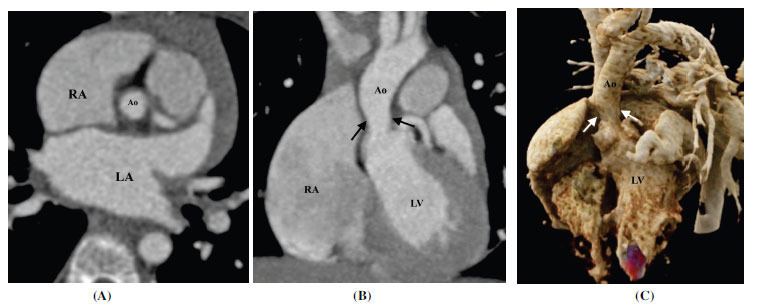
Supravalvular aortic stenosis. (A) Axial contrast-enhanced computed tomographic angiography image showing the narrowed caliber of the supravalvular aorta (B) Coronal contrast-enhanced computed tomographic angiography image and (C) Volume rendered technique image showing supravalvular aortic stenosis (arrow). Abbreviations: RA; right atrium, LA; left atrium, LV; left ventricle, Ao; ascending aorta.
